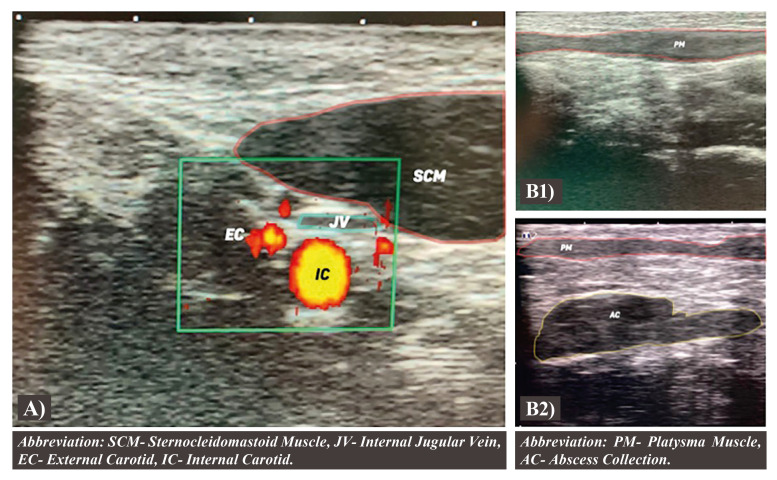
A) Ultrasonography image with doppler enhanced study of the carotid bifurcation region revealing the sternocleidomastoid, internal jugular vein, and internal and external carotid. B) Ultrasonography image of the submandibular area, left side normal and right side affected. It is necessary to assess the relationship between the platysma and the adjacent structures. On the affected side, an abscess collection is observed, distorting the local morphology.
